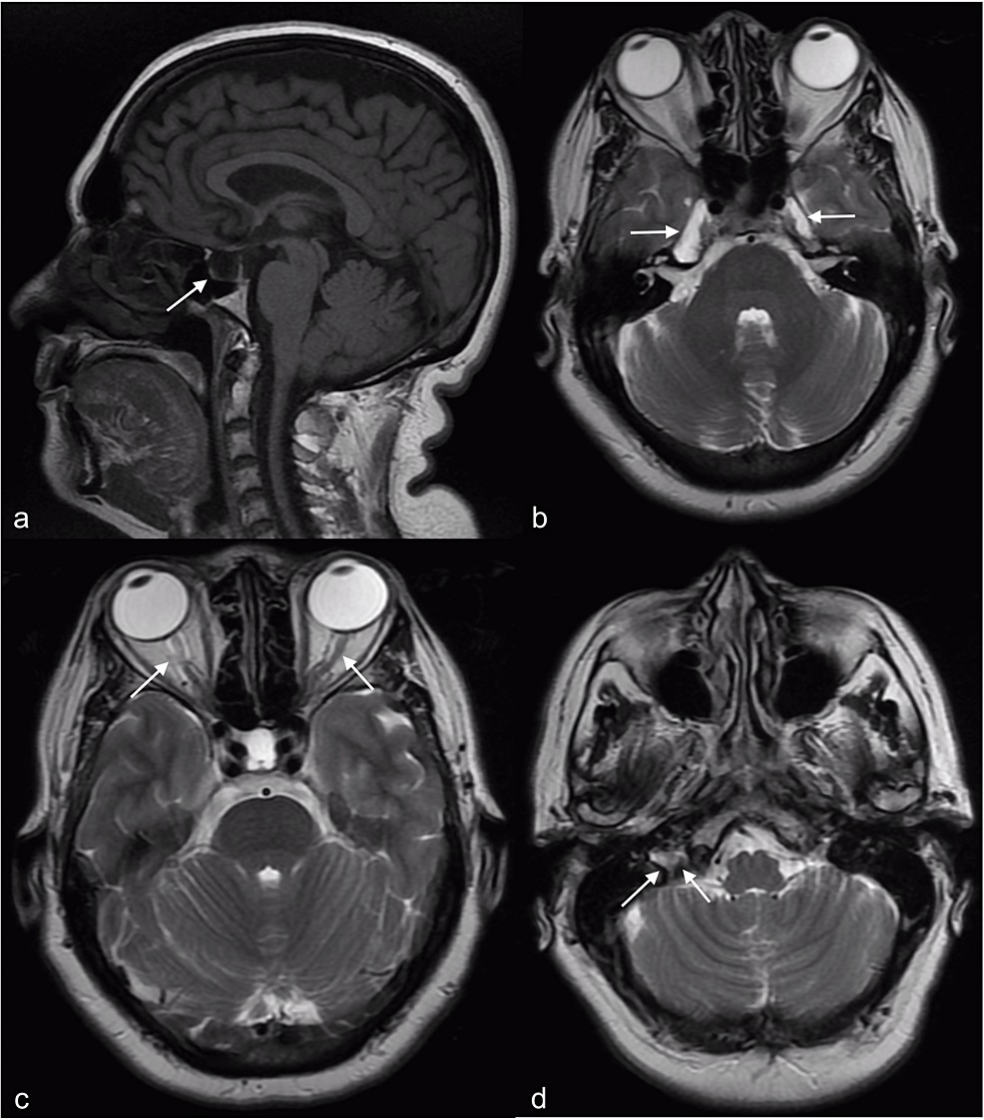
Magnetic resonance imaging of the brain shows indirect signs of intracranial hypertension. (a) Sagittal view showing empty Sella turcica (white arrow). (b) Axial view showing dilation of Meckel's cavum (white arrows). (c) Axial view showing thickening of the optic nerve sheath (white arrows). (d) Axial view showing chronic occlusion of the right internal jugular vein (white arrows).
